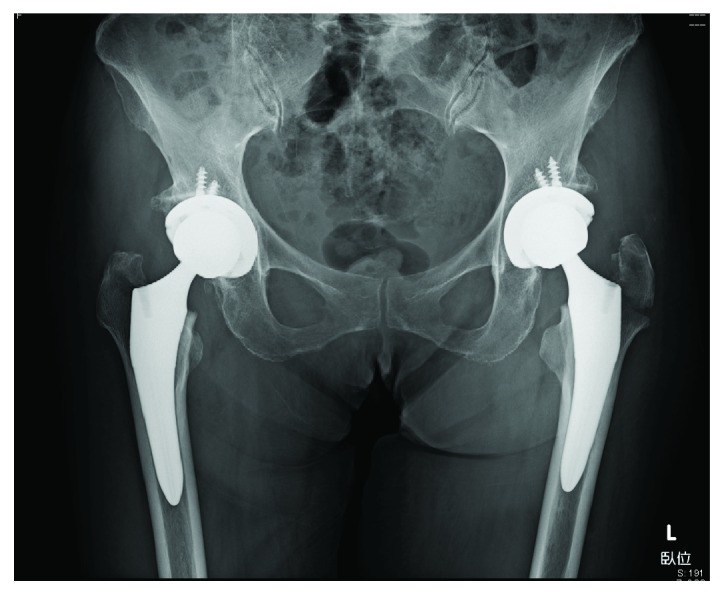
On the 14th postoperative day, the patient complained of left coxalgia during a walking exercise without any falls. Plain radiographs revealed a left greater trochanteric avulsion fracture.
